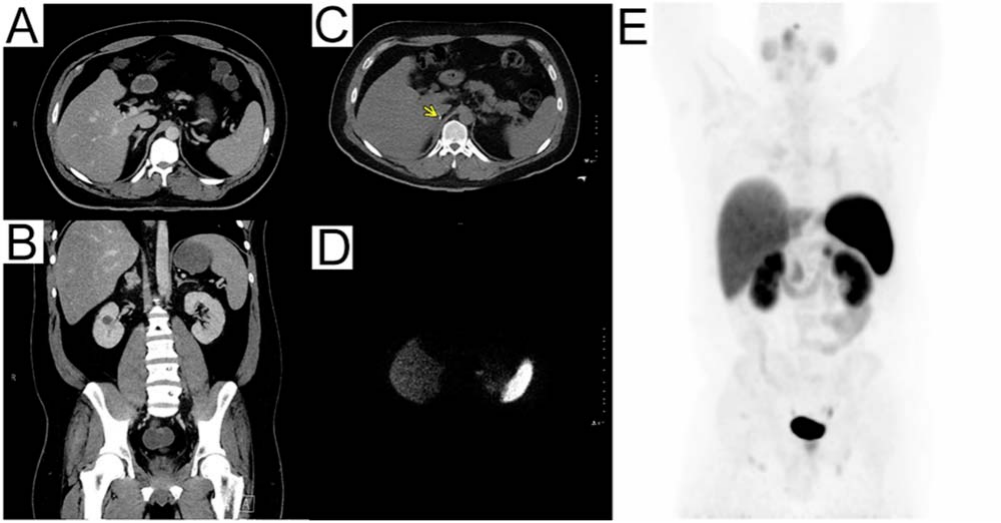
(A) Axial contrast-enhanced CT image demonstrates a 2.8 cm right adrenal mass with irregular contours and a heterogeneous enhancement pattern. (B) Coronal CT image further highlights the heterogeneity of the adrenal mass and reveals multiple sub-centimeter renal cysts. (C) Axial postoperative CT image shows postsurgical changes in the right adrenal bed; the arrow denotes a surgical clip in the adrenal fossa with no evidence of recurrent mass. (D) The corresponding axial ^68^Ga-DOTATATE PET image at the same level demonstrates no abnormal somatostatin receptor-avid uptake in the adrenal bed. (E) Whole-body ^68^Ga-DOTATATE PET maximum intensity projection (MIP) image shows no evidence of residual or metastatic disease.
